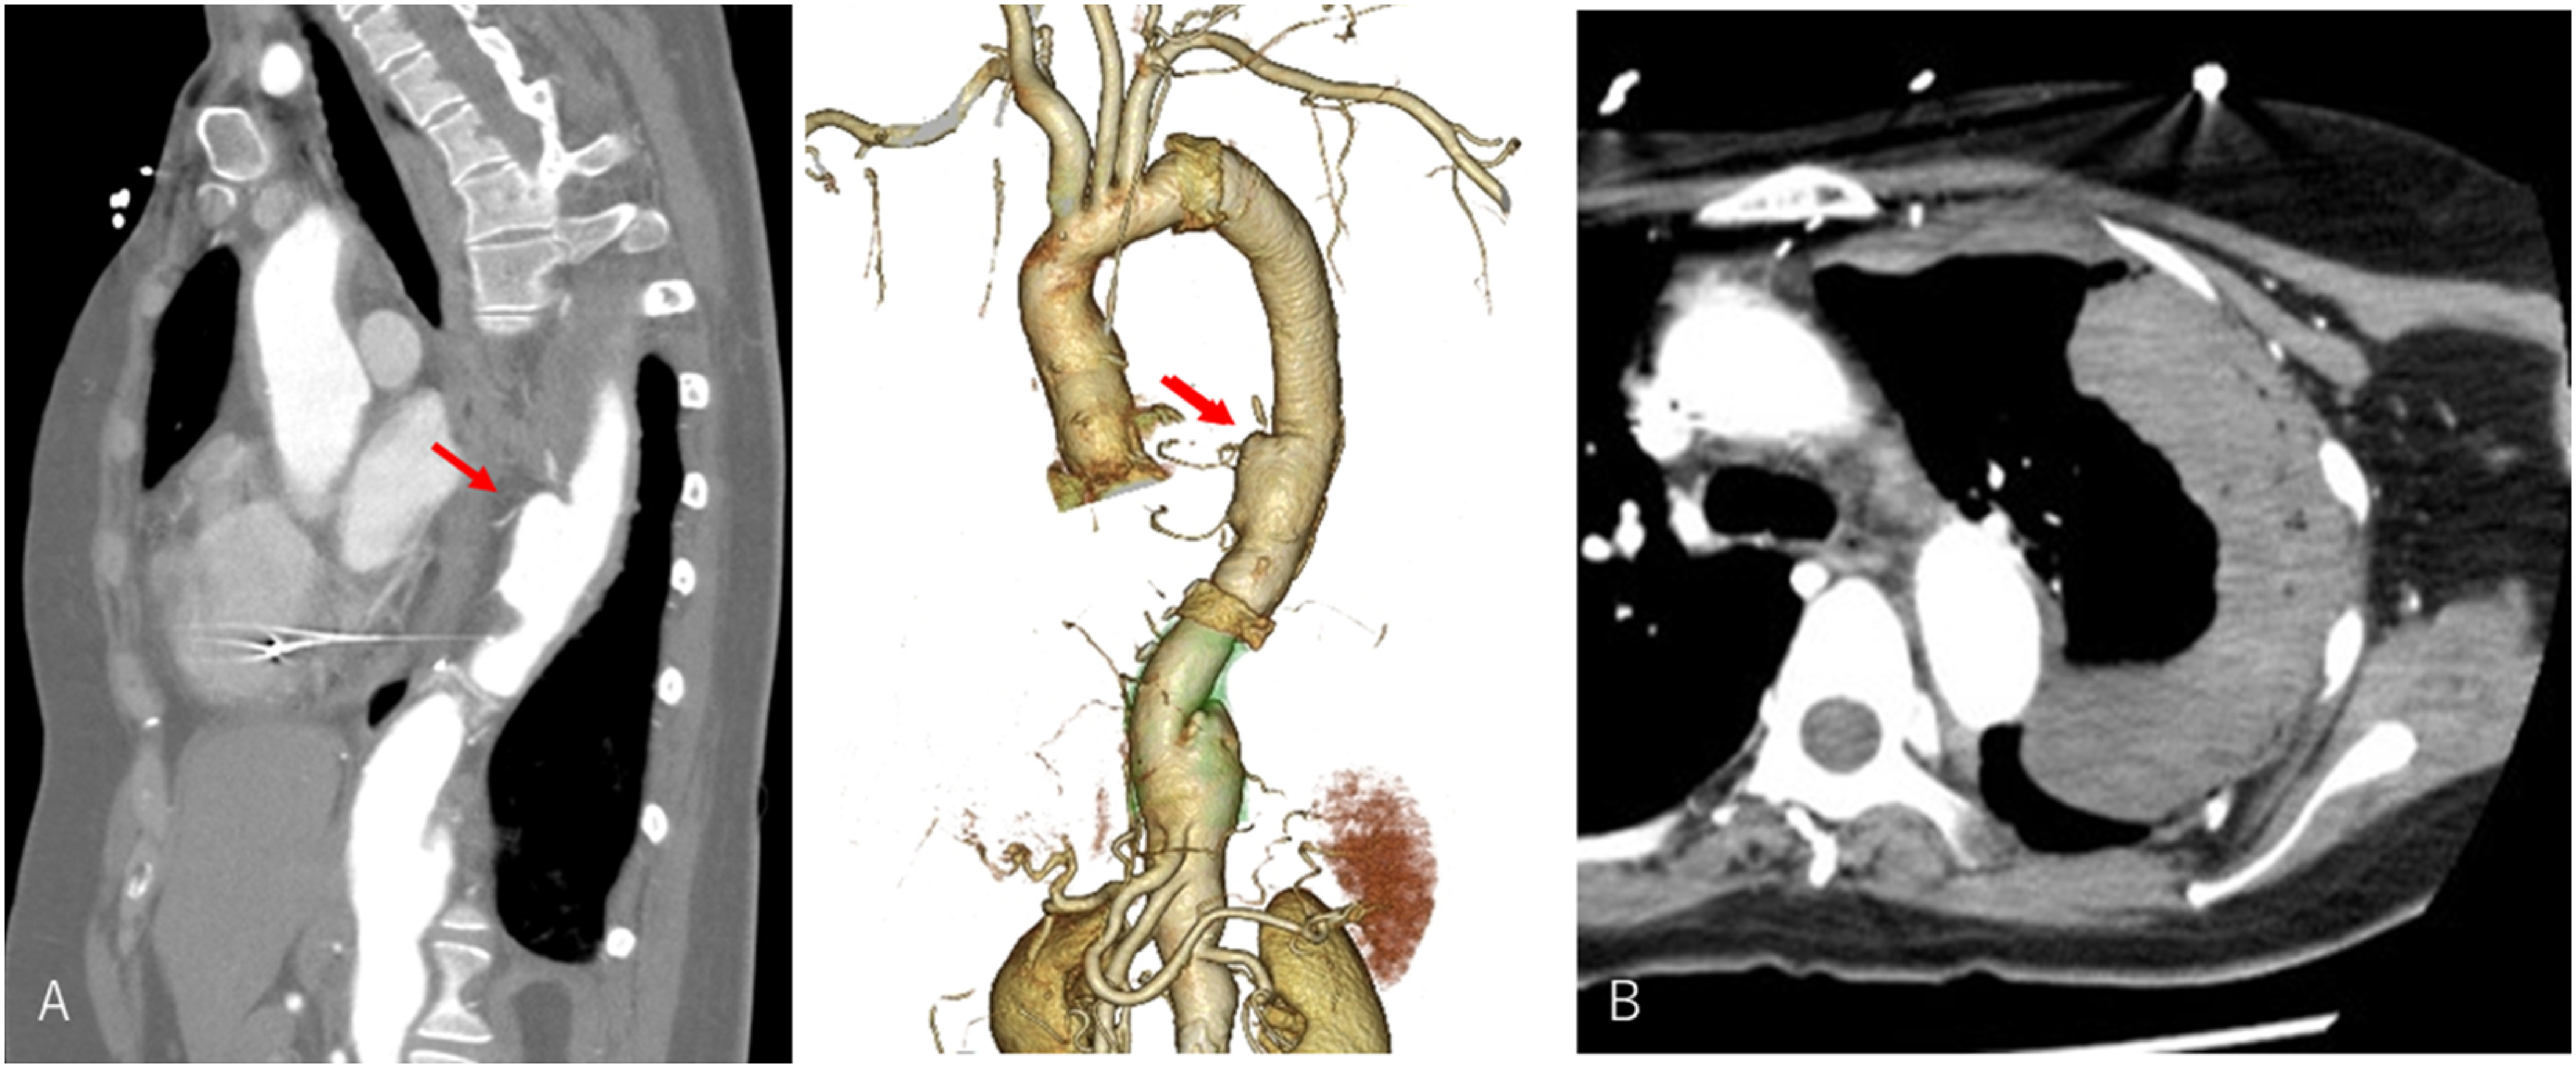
Fig. 1 (A) Contrast enhanced computed tomography image showing the intercostal patch aneurysm 32-mm in diameter (arrow). (B) The hematoma was separated from the aneurysm and accumulated in the apex of the lung.
